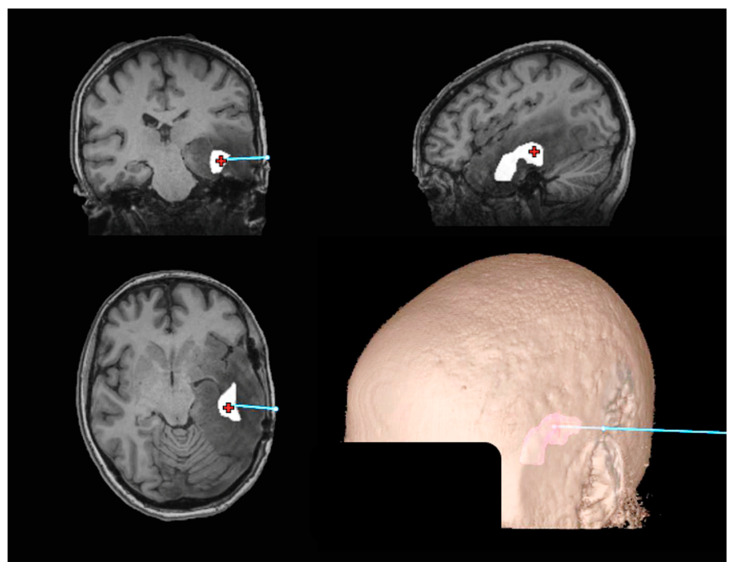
Medtronic S8 Stealth^®^ Navigation Tool (Medtronic, Minneapolis, MN, USA) with the 5x Cho/NAA biopsy target displayed. The entry point coordinate was (72, 15, −14), and the target point, depicted by the red crosshair, was at coordinates (36, 12, −15) in the MRI DICOM space. The total travel distance was 36.1 mm to reach the target volume. The blue line shows the projected travel of the needle. The patient’s face is obscured for anonymity.
